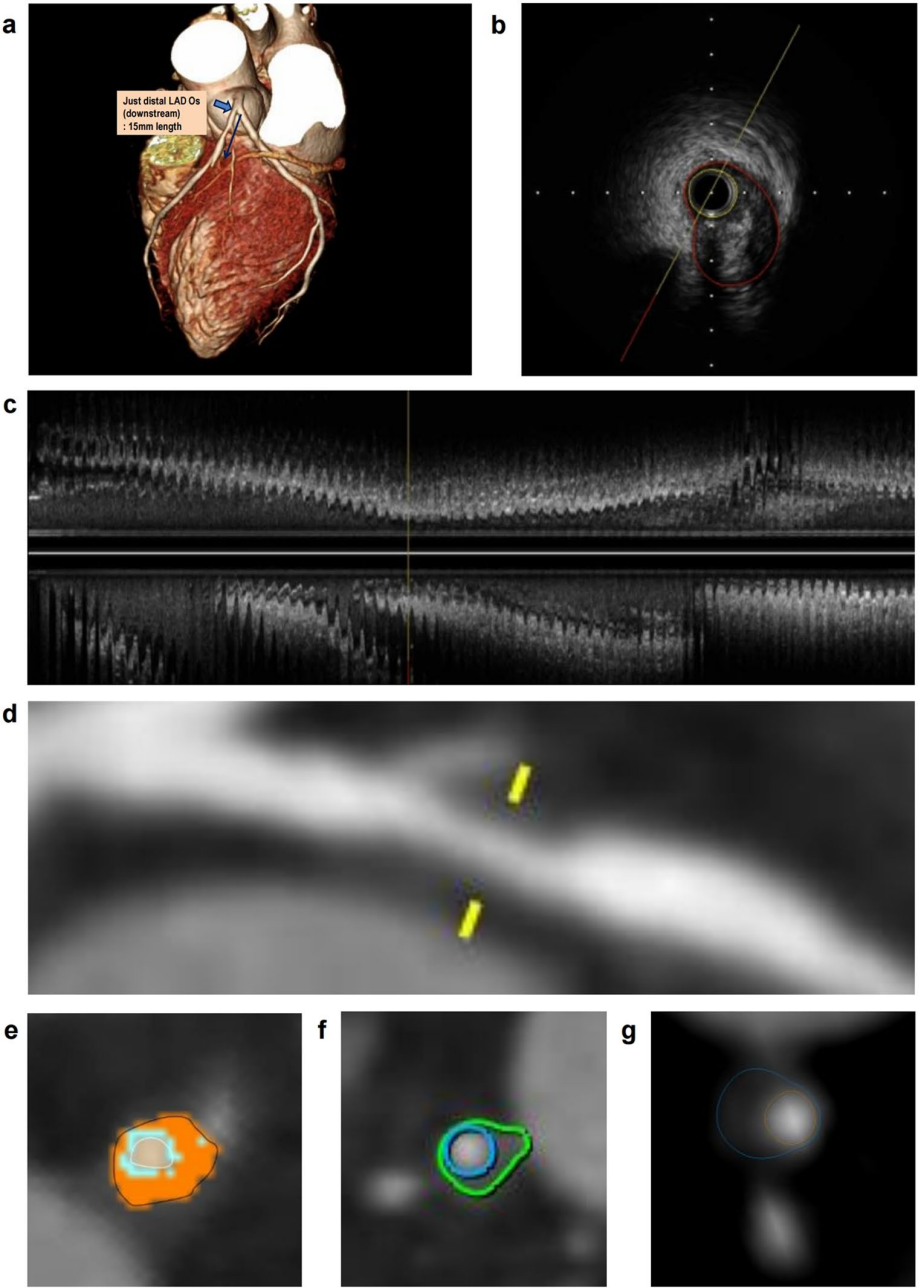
Representative images of the curved planar reformation and cross-sectional planes of the lumen or plaque region for each software platform. (a) The location information annotated on a three-dimensional image of the coronary computed tomography angiography that provided the reference to the exact length of the target segment. (b) The representative cross-sectional plane of the IVUS. (c-d) Longitudinal and curved planar reformation images of the target segment of the IVUS (c) and the software 1 (d), respectively. (e-g) The representative cross-sectional planes for software 1 (e), 2 (f), and 3 (g). Inner and outer lines (white and black for software 1; blue and green for software 2; orange and blue for software 3) represent the lumen and vessel boundaries, respectively. Software 1 = AutoSeg, AI Medic; Software 2 = Syngo.via, Siemens; Software 3 = IntelliSpace Portal, Philips
